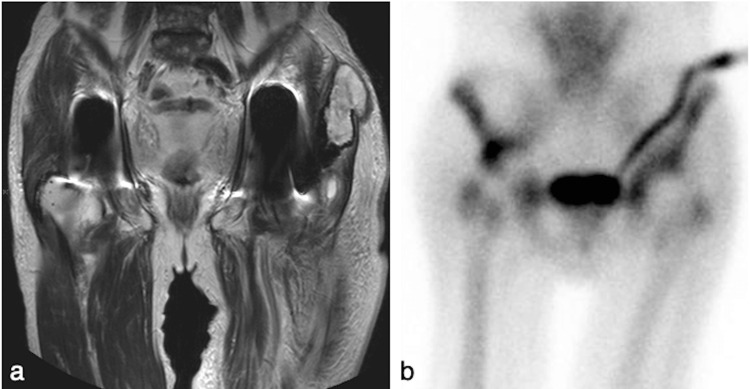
Preoperative imaging. (a) T2-weighted magnetic resonance imaging showed a pseudotumor in the left hip. (b) Bone scintigraphy showed uptake in both hips.
